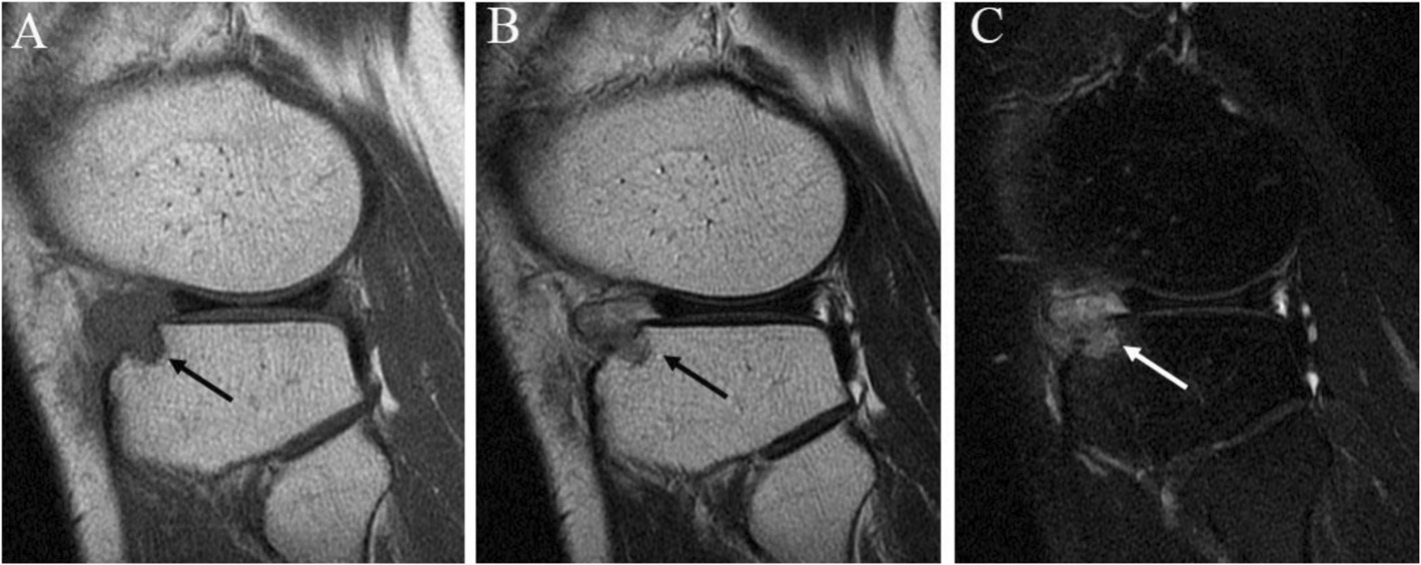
Magnetic resonance imaging reveals an intra-articular mass (arrow) in the knee joint. (A) T1-weighted magnetic resonance imaging reveals that the mass is homogeneous and isointense to normal muscle. (B) and (C) T2 with saturated fat signal sagittal section images show heterogeneous signal intensity with specks. Magnetic resonance imaging: Discovery-MR750 3.0 Tesla (GE Healthcare, Waukesha, WI, USA); T1: repetition time/echo time = 444/10, T2: repetition time/echo time = 3500/90, T2 saturated fat signal: repetition time/echo time = 4400/102, field of view = 160×160mm, slice thickness = 4mm.
